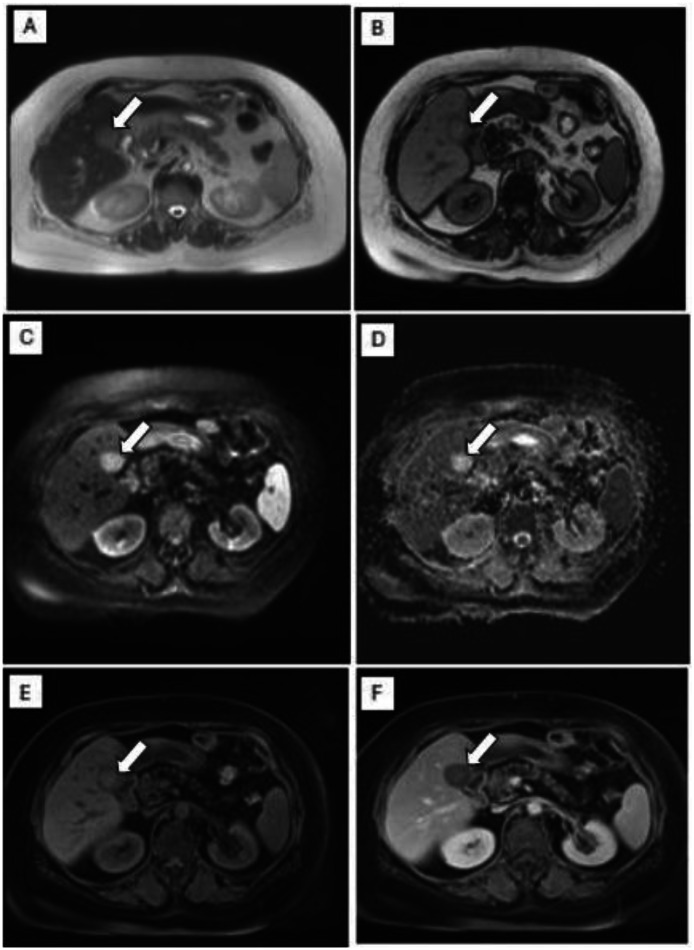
MR images of a patient with a postcholecystectomy lesion located in the cholecystectomy bed, indicated by the white arrow on all images. The lesion (white arrow) demonstrates intermediate signal intensity on T2-weighted SS-FSE images (A) and low-to-intermediate signal intensity on T1-weighted opposed-phase images (B). The lesion marked by the white arrow appears hyperintense on both DWI (C) and ADC maps (D), consistent with the absence of diffusion restriction. On noncontrast T1-weighted imaging (E), the lesion identified by the white arrow shows no intrinsic hyperintensity, and no enhancement is observed on postcontrast T1-weighted images (F).
